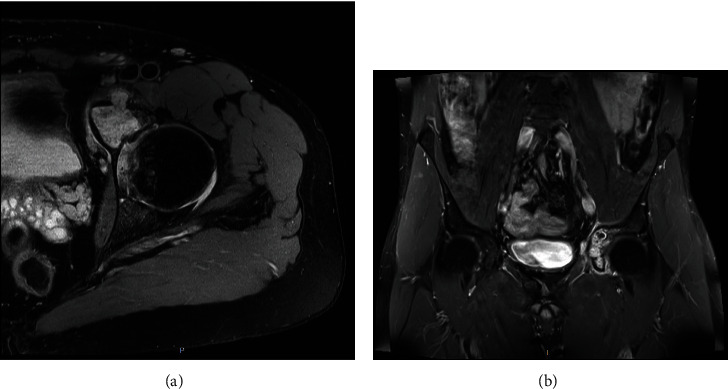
Axial (a) and coronal (b) T1 TSE sequence views on initial MRI of the pelvis showing a tumor of the left iliopubic ramus.
